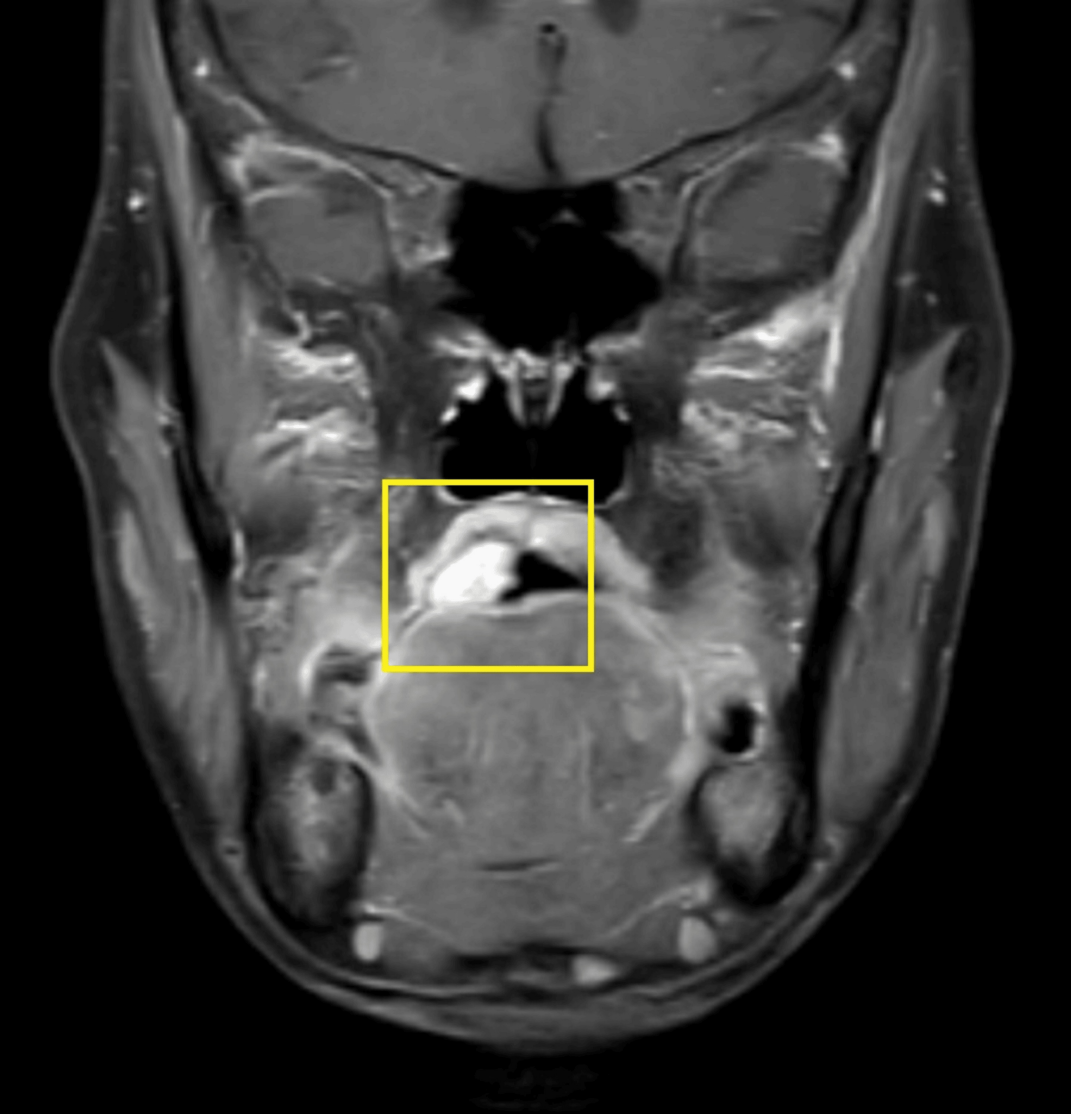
MRI of the oral cavity (coronal view) MRI: magnetic resonance imaging
